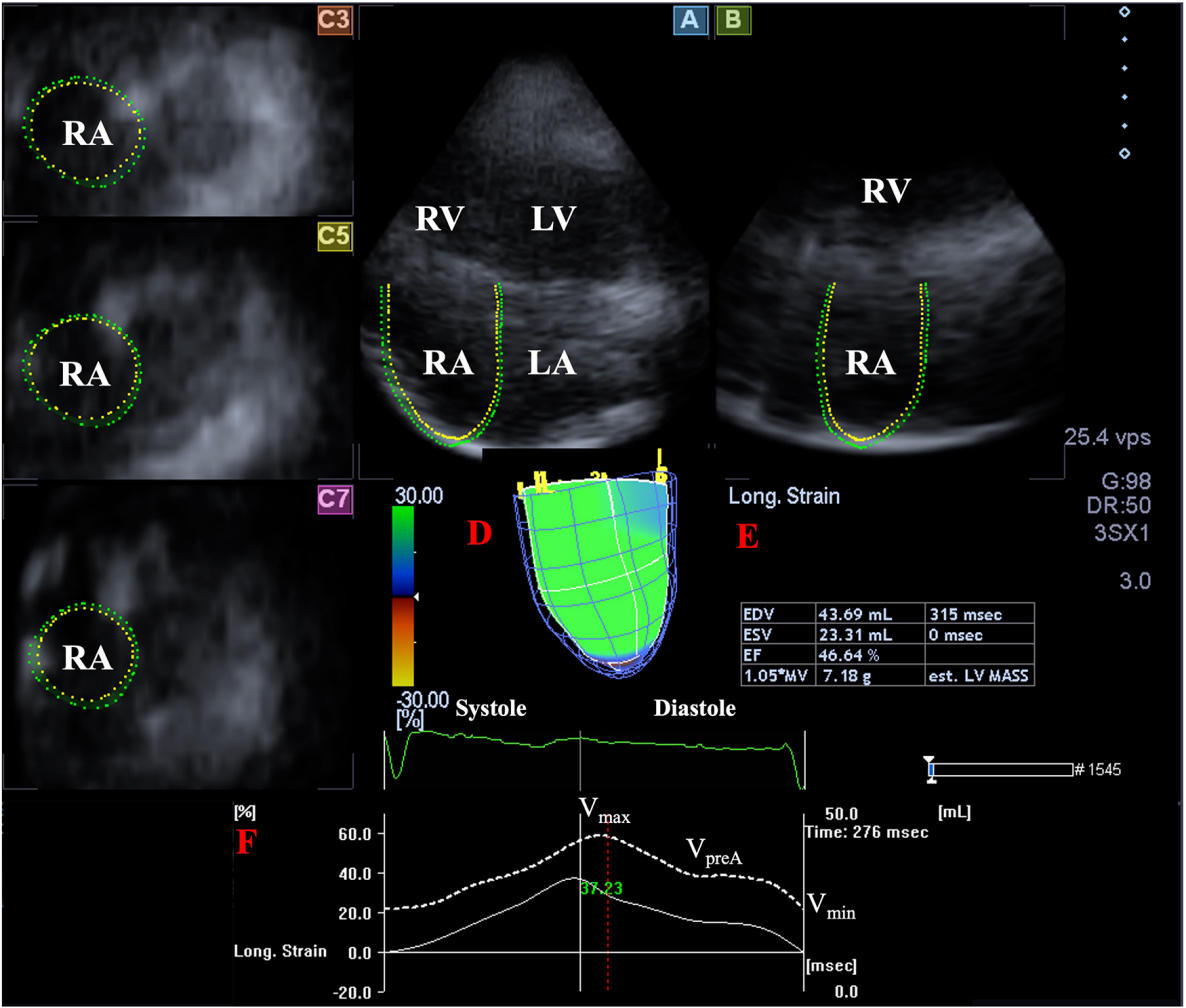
Three-dimensional (3D) speckle-tracking echocardiographic analysis of the right atrium in a healthy subject: apical longitudinal four-chamber (A) and two-chamber views (B) and 3 short-axis views at basal (C3), midatrial (C5) and superior (C7) RA levels. 3D virtual RA model (D), RA volumetric data (E) and time—global RA volume change curve (dashed white curve) and time—global RA longitudinal strain curve (white curve) respecting the cardiac cycle are demonstrated (F). LA, left atrium; LV, left ventricle; RA, right atrium; RV, right ventricle; EDV, end-diastolic volume; ESV, end-systolic volume; EF, ejection fraction; est., estimated; MV, myocardial volume; V_max_, maximum right atrial volume; V_preA_, volume at the onset of atrial systole; V_min_, minimum right atrial volume.
